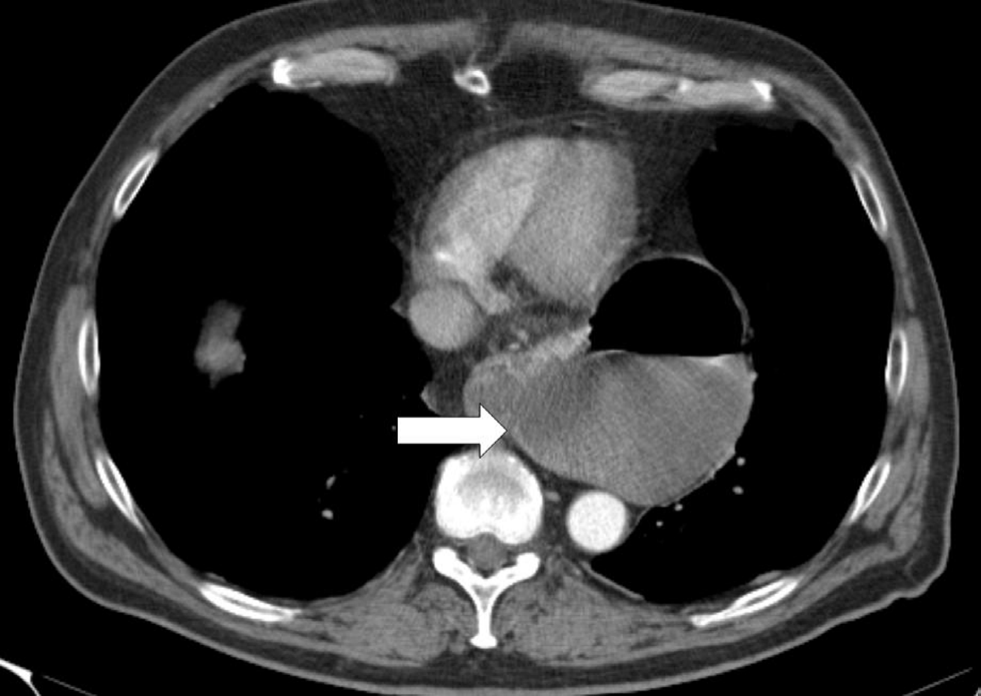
Preoperative computed tomography of the upside-down stomach of a 73-year-old male with the gastroesophageal junction being fully dislocated into the thoracic cavity (arrow).
